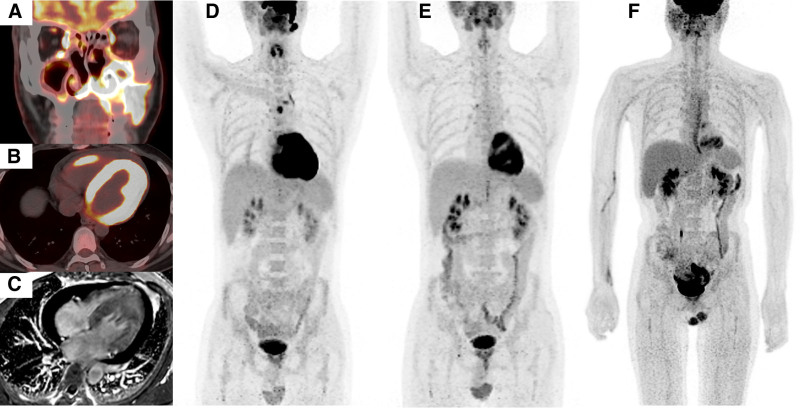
Representative images of cardiac and primary lesion. (A and B) PET/CT fusion images: lesions with increased glucose metabolism in the nasal and facial regions (SUVmax: 32.1), increased local glucose metabolism in the right ventricular wall, left ventricular wall and interventricular septum (SUVmax:37.8). (C) CMR imaging: Mild thickening of the left ventricle and interventricular septum, with delayed enhancement of the left ventricular wall. (D) PET MIP: increased local glucose metabolism in heart and Mediastinal lymph nodes have been showed. (E) PET MIP after 2 cycles of chemotherapy: The range of nasal-facial lesions was reduced with a decrease in glucose metabolism (SUVmax: 6.2), and a significant decrease in glucose metabolism in mediastinal lymph nodes and the ventricular wall (SUVmax: 12.7). (F) PET MIP after 5 cycles of chemotherapy MIP: the nasal-facial and mediastinal lesions have completely relieved, and myocardial glucose metabolism has decreased compared to the previous time. CMR = cardiac magnetic resonance, MIP = maximum intensity projection, PET = positron emission tomography.
